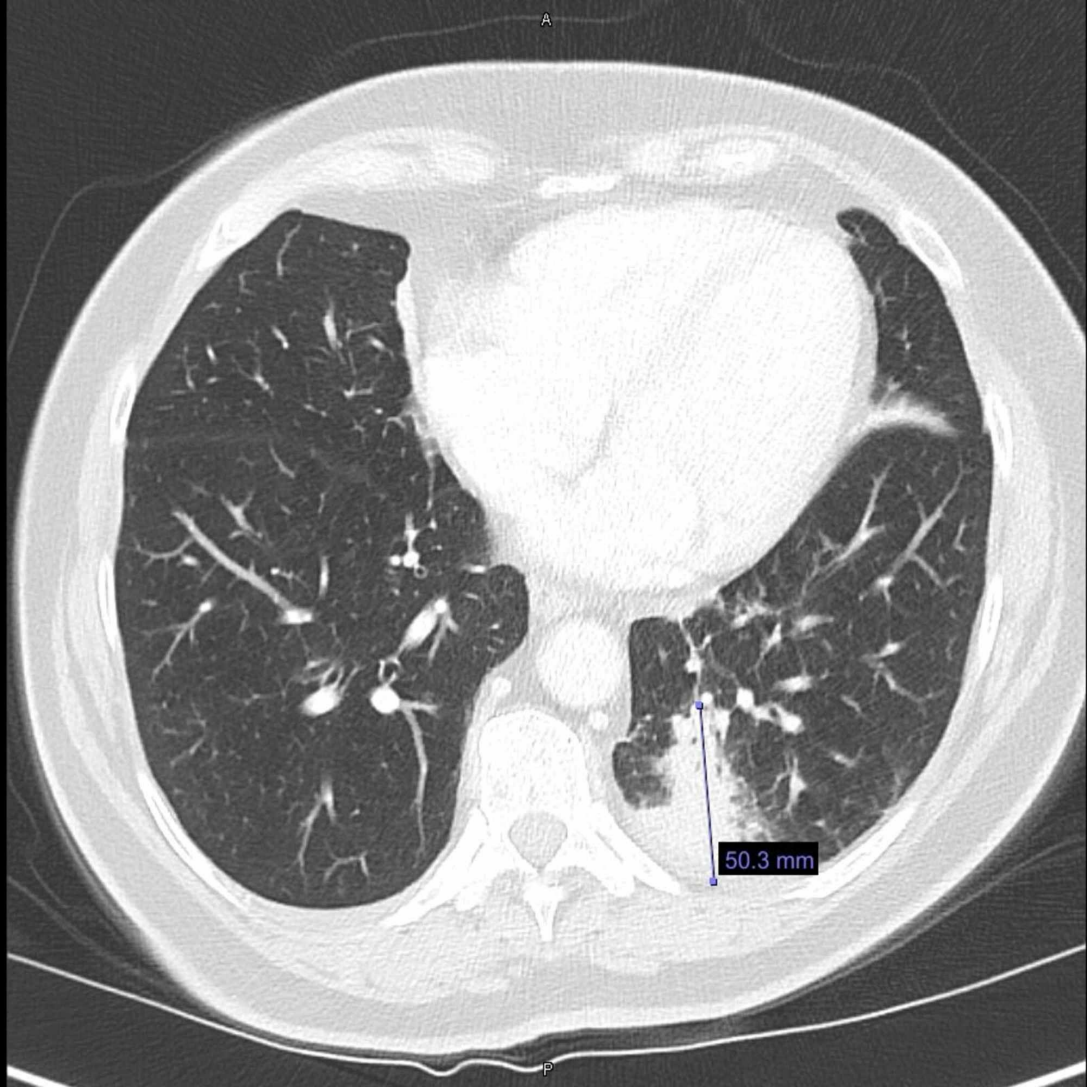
A CT scan of the chest further shows a 50.3-mm mass in the lower lobe of the left lung along with an enlarged left-sided mediastinal lymph node.
